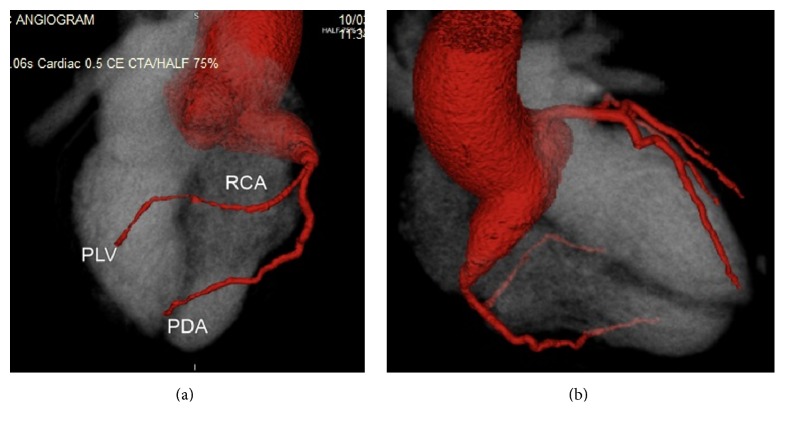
CT coronary angiography demonstrating right coronary sinus of Valsalva. Aneurysm viewed (a) anteriorly and (b) posteriorly.
